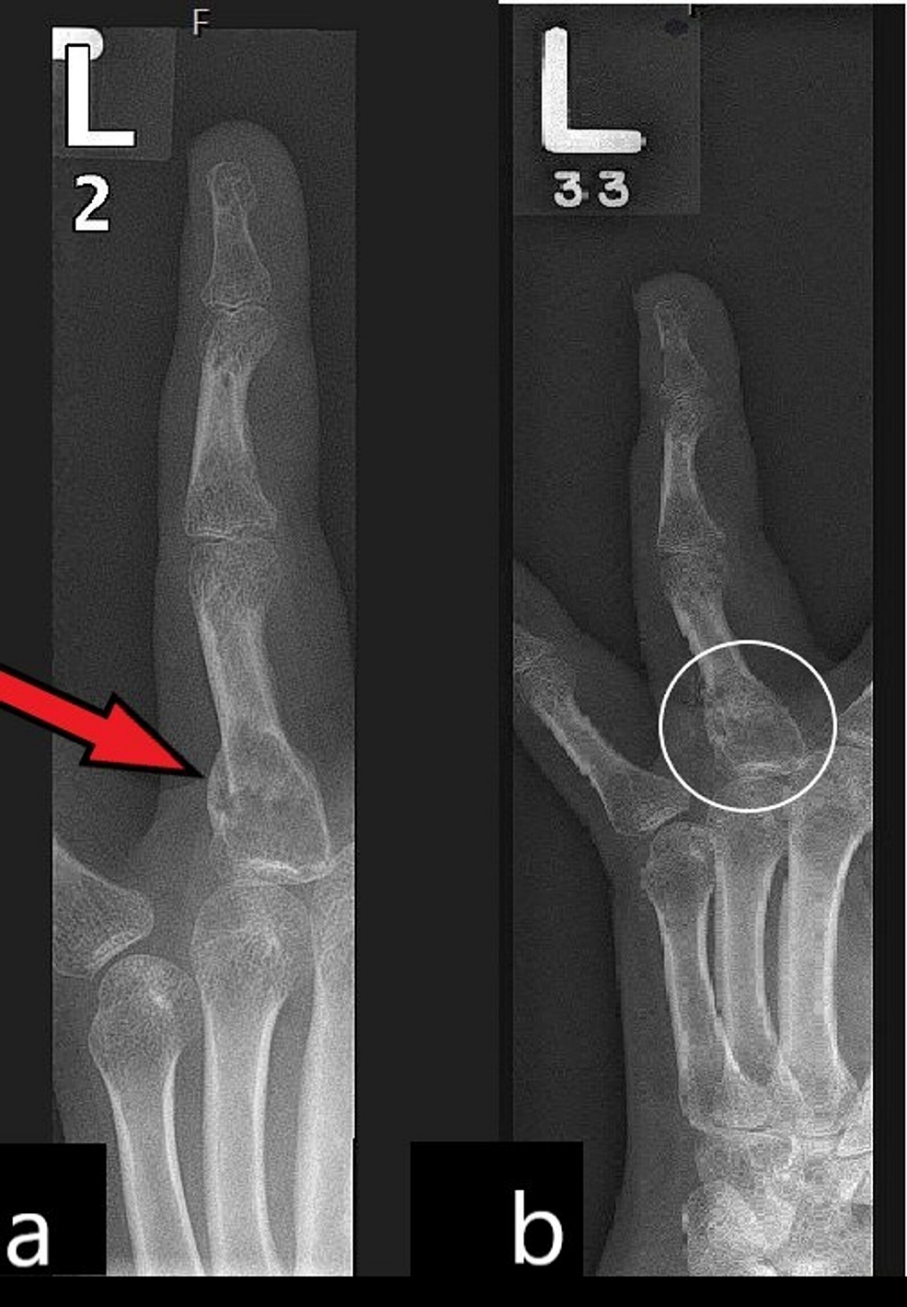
Follow-up radiographs of the left ring finger at two months showing (a) consolidation of the fracture at the base of the proximal phalanx (red arrow) and (b) almost complete bony union at six months (white arrow). The lytic lesion persists with sclerosis in the distal margin at the base of the proximal phalanx.
